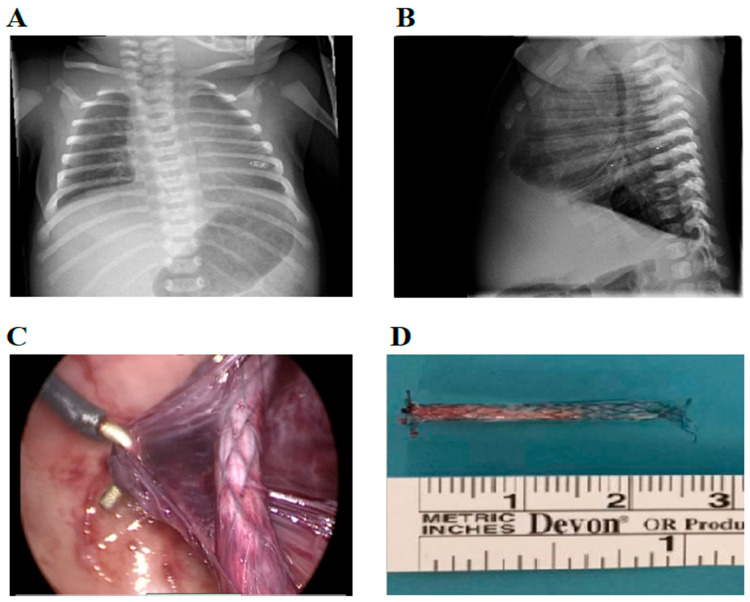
Postnatal chest radiography and surgical view of shunt removal via thoracoscopy. (A) Chest X-ray showing the shunt located inside de left hemithorax. (B) Lateral view showing costophrenic angle blunting revealing pleural effusion. (C) Thoracoscopic adhesiolysis and removal of device. (D) Somatex^®^ shunt outside the thoracic cavity showing its 25mm length, self-deploying parasols and nitinol wire mesh.
